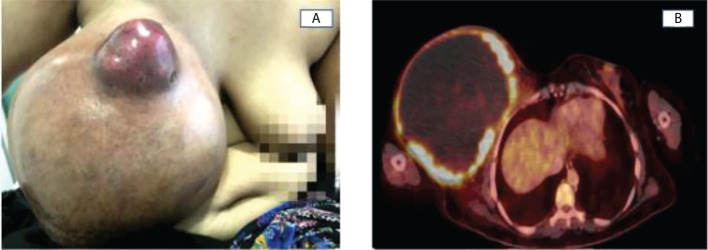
(A) Enlarging right breast cancer with a painless, ulcerated, hard, irregular mass in the union of the upper quadrants. (B) Voluminous hypermetabolic mass of 18.4x15.6cm, with a cystic component in the right breast and a SUVmax >4.
